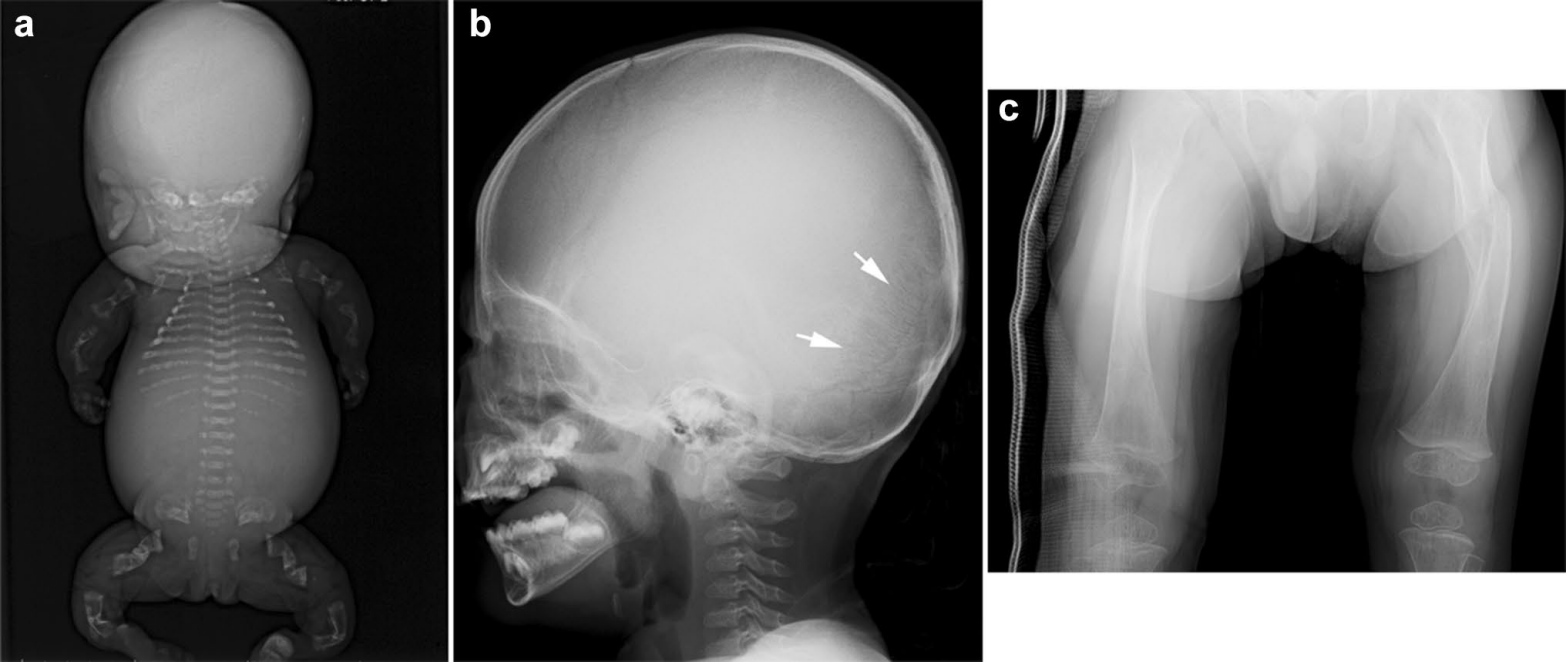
a A stillborn with osteogenesis imperfecta. Radiograph shows a beaded appearance of the ribs and an “accordion-like” wavy appearance of the long bones as a consequence of in utero multiple fractures. b, c A 2-year-old patient with osteogenesis imperfecta. Radiographs show multiple Wormian bones (arrow) due to defective calvarial ossification and generalized osteoporosis with a healing fracture of the right femoral shaft and a healed fracture of the left femoral shaft
